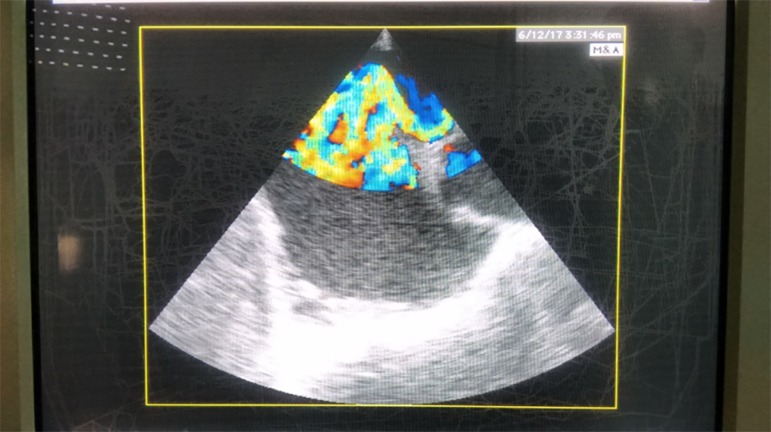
Snapshot during second transesophageal echocardiography examination
